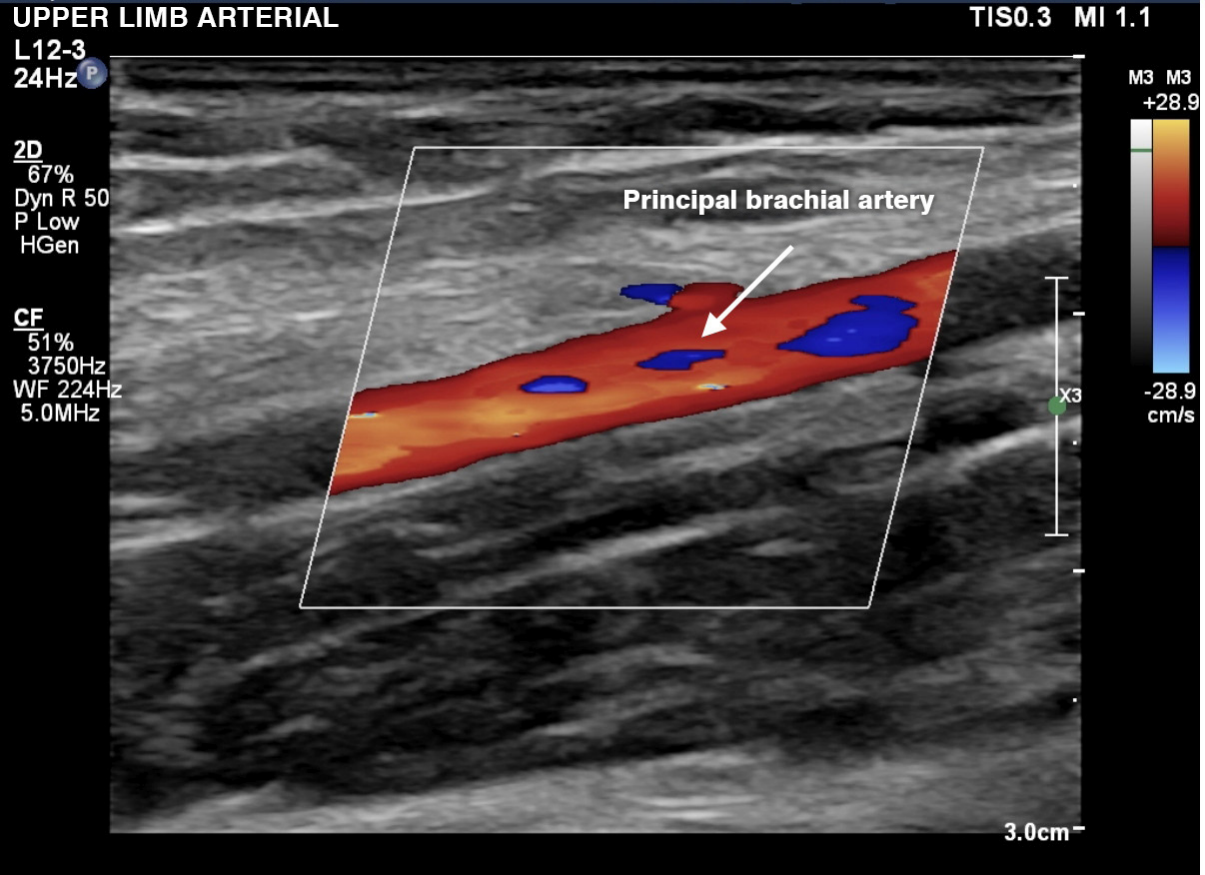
Doppler ultrasound in longitudinal view, showing the principal brachial artery
